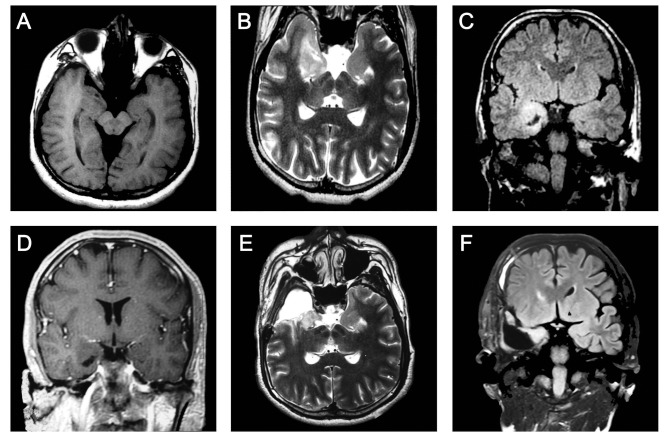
(A and B) Pre-surgery T1- and T2-weighted images show an approximate 2.0×1.4 cm region of abnormal signal in the right anterior and medial temporal lobe (long T1 and T2 weighted signal). (C) T2-weighted and FLAIR images showed a reduced hippocampal volume with relatively increased signal on the right side and slightly enlarged temporal horn, which are typical findings of HS and FCD. (D) Contrast-enhanced MRI showed no signs of enhanced signals. (E and F) Post-surgery T1- and T2-weighted images showed partial lesion residual in right temporal lobe. HS, hippocampal sclerosis; FCD, focal cortical dysplasia.
